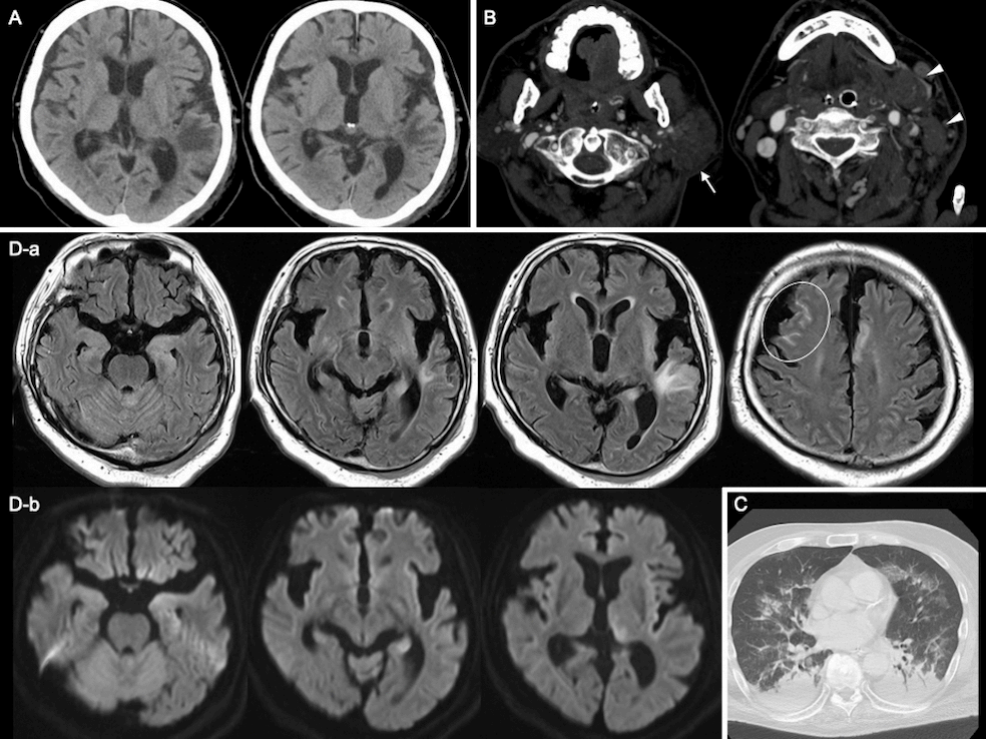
Images *Computed tomography (CT) images* A) Head CT image revealed a low-intensity area at the left temporal lobe. B) Cervical CT imaging showed lymphadenopathy from the left neck to the left axilla (arrow heads) and a continuous mass lesion in the left parotid glands (arrow). C) Chest CT imaging showed bilateral pleural effusion and passive atelectasis, and infiltrative shadows in the bilateral upper lobe, middle lobe, and lingular segment. *Magnetic resonance (MR) images* D-a) Fluid-attenuated inversion recovery imaging of the brain revealed a high intensity in the left hippocampus, insula, and cingula, and edematous change of the left temporal lobe. Hyperintensity in the subarachnoid space of the right frontal lobe suggesting meningeal carcinomatosis (circle). D-b) Diffusion-weighted imaging signal hyperintensity in the left pulvinar of the thalamus and hippocampus. These findings suggested NCSE.
